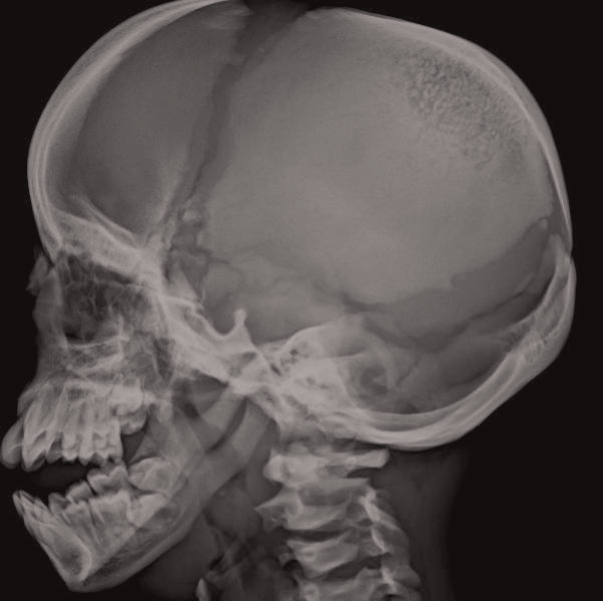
Skull X-ray showing obtuse angled mandible, open anterior and posterior fontanelle and non pneumatised sinuses.
